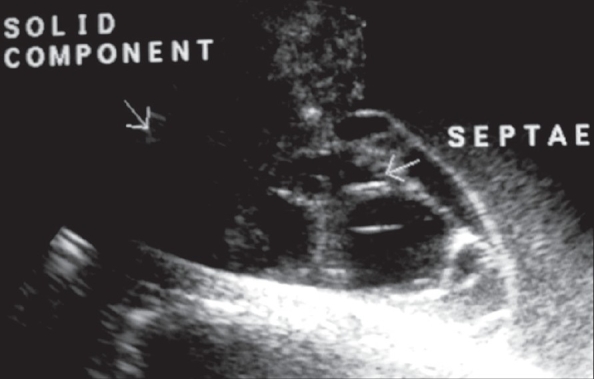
Transvaginal sonography showing a complex cystic mass with multiple internal septations in the right adnexal region
